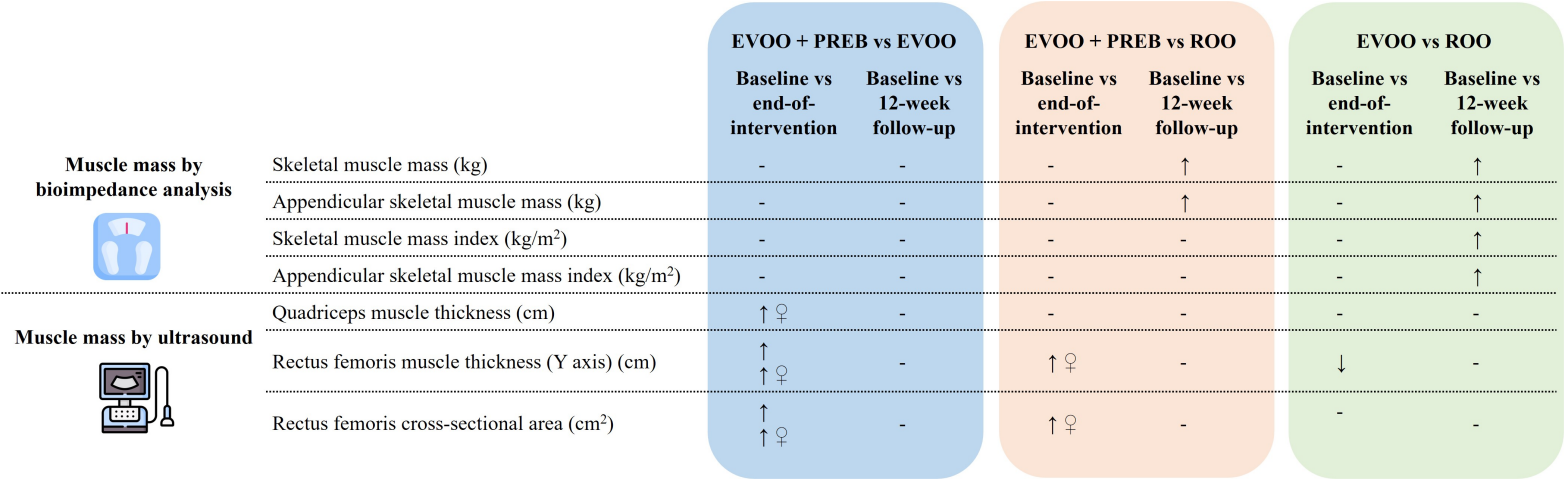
Muscle mass results assessed by BIA and ultrasound. EVOO + PREB; extra virgin olive oil rich in phenolic compounds and prebiotic group; EVOO: extra virgin olive oil rich in phenolic compounds and maltodextrin placebo group; ROO: refined olive oil and maltodextrin placebo group. Related to muscle mass assessed by BIA at 12‐week follow‐up, a significant increase in SM and ASM was shown in the EVOO + PREB group and EVOO group compared to the ROO group in all population. Additionally, at the 12‐week follow‐up, a significant increase was observed in SMI and ASMI in the EVOO group compared to the ROO group in all population. Concerning ultrasound parameters, for quadriceps MT at the end‐of‐intervention, a significant increase was shown in the EVOO + PREB group compared with the EVOO group in the female population. Related to the rectus femoris MT (Y axis) at the end‐of‐intervention, a significant increase was observed in the EVOO + PREB group compared to the EVOO group in all population and females. Also, at the end‐of‐intervention, a significant increase was shown for rectus femoris MT (Y axis) in the EVOO + PREB group compared to the ROO group in the female population. Moreover, at the end‐of‐intervention, rectus femoris MT (Y axis) significantly decreased in the EVOO group compared to the ROO group in all population. For rectus femoris CSA at the end‐of‐intervention, a significant increase was shown in the EVOO + PREB group compared with the EVOO group in all population and females. Also, at the end‐of‐intervention, a significant increase was shown in the EVOO + PREB group compared to the ROO group in females.
